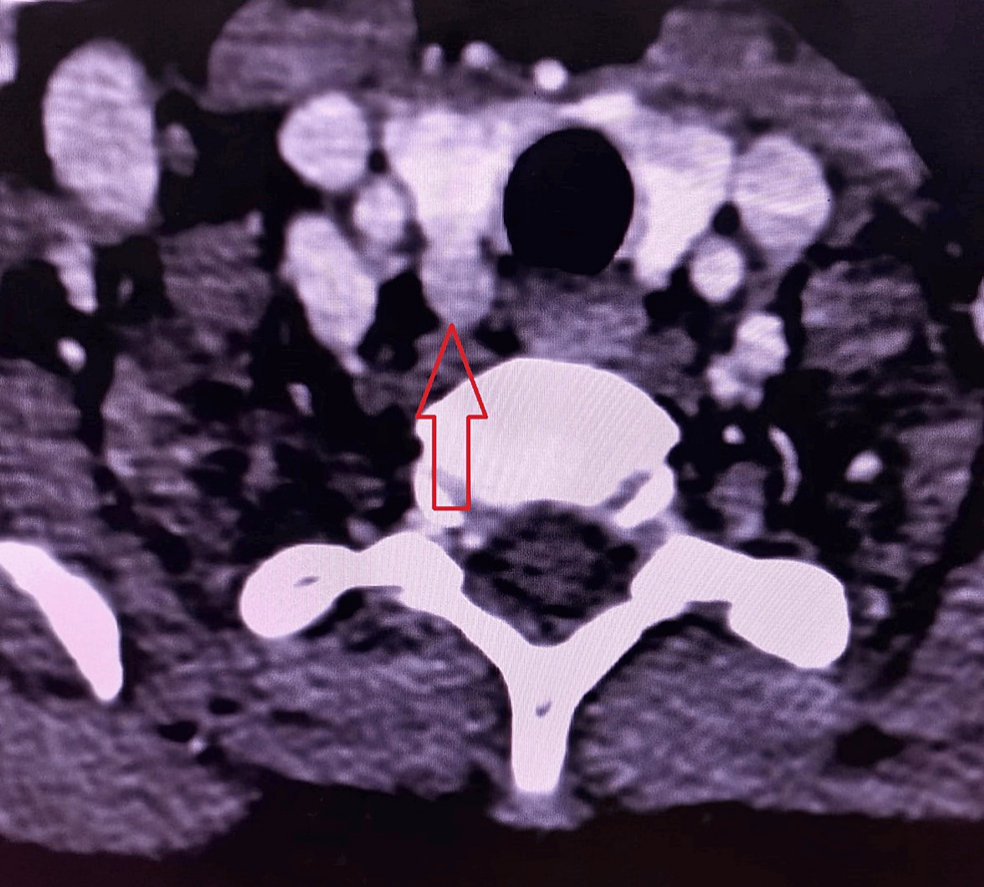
4D-CT neck showing right inferior parathyroid adenoma
